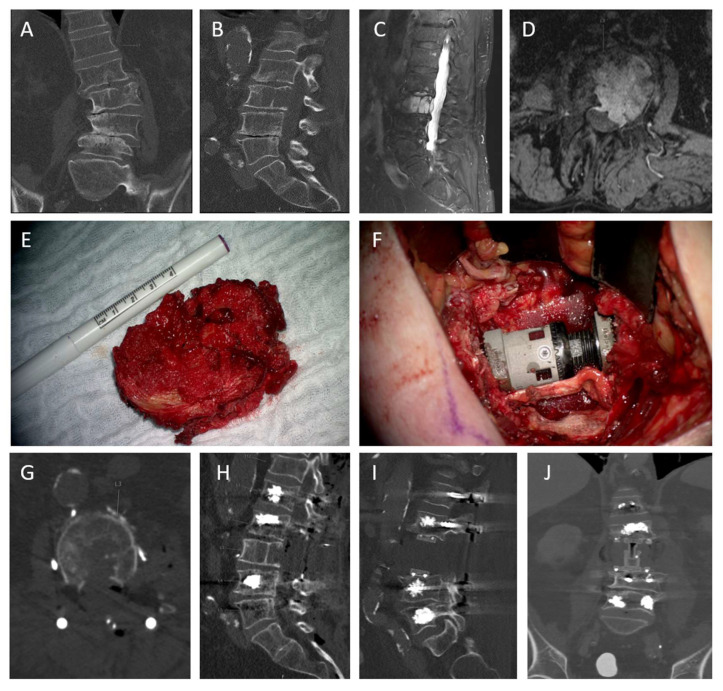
Pre- and postoperative imaging of a case of a chordoma of L3. (A,B): preoperative coronal and sagittal CT showing osteolysis and thoracolumbar scoliosis (C,D): sagittal and axial T1 contrast-enhanced MRI imaging showing the extent of the tumor (E): intraoperative photograph showing en bloc removed vertebra (F): intraoperative photograph showing carbon PEEK implant (G,H): CT axial and sagittal after first surgery (dorsal instrumentation, cementaugmentation, dorsal release) (I,J): sagittal and coronal CT after second step showing carbon implant according to [15].
